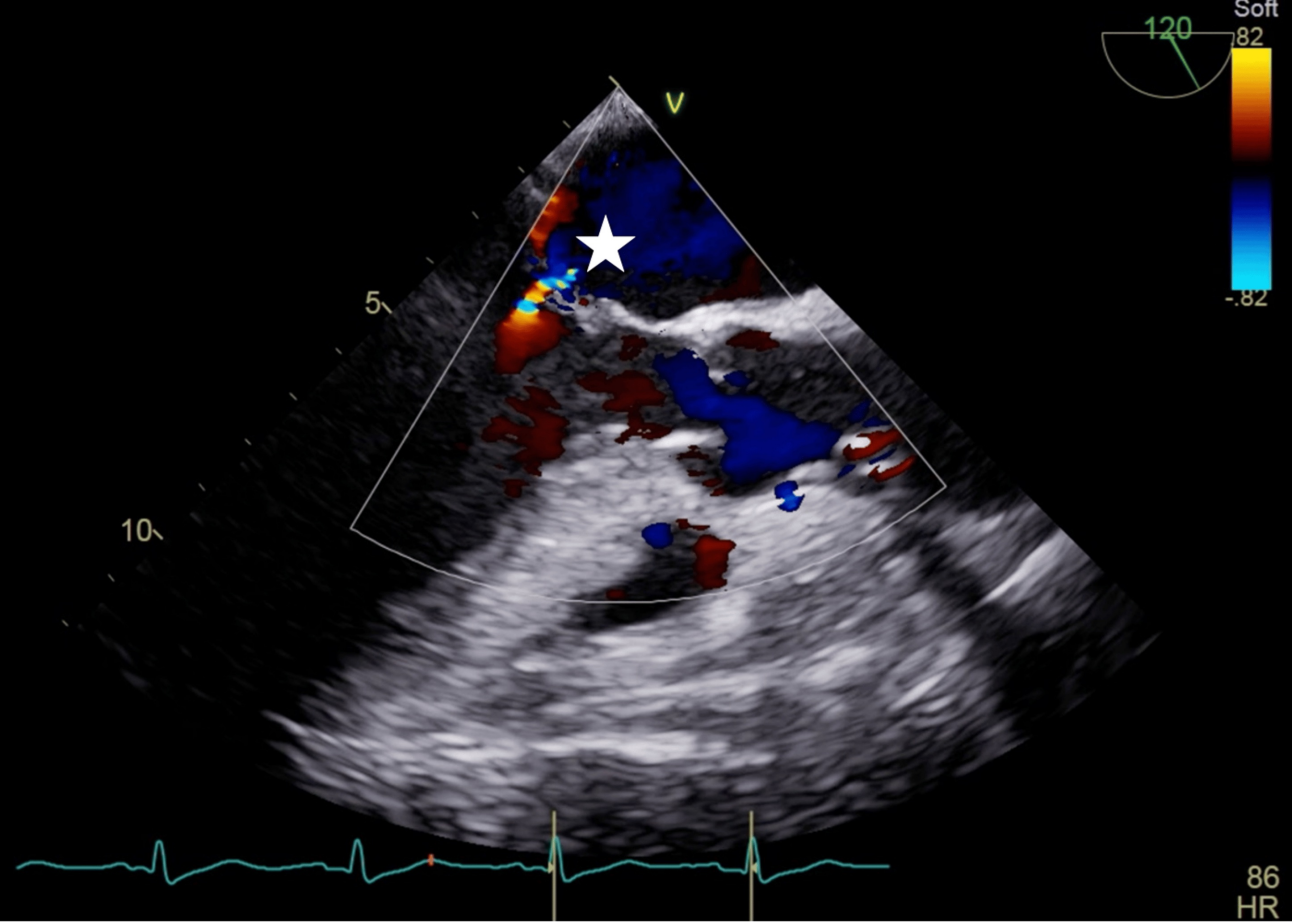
TEE findings after anesthetic induction. The LVEF was 40%, and there was a mild hypokinesis of the left ventricular motion and mild mitral regurgitation flow centrally from the commissure of the mitral valve (asterisk). TEE: transesophageal echocardiography; LVEF: left ventricular ejection fraction
